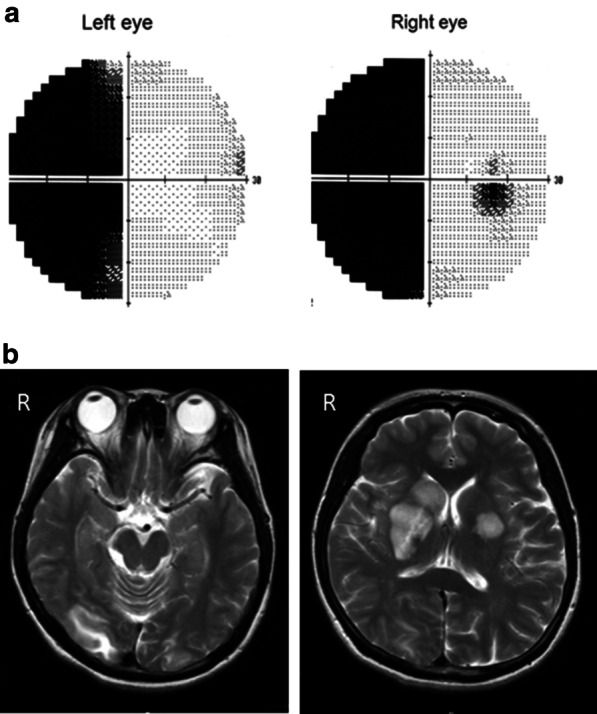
Visual field and magnetic resonance imaging before treatment. a Left homonymous hemianopsia was revealed. b MRI at 3 days after onset of the severe headache. T1-weighted-fluid-attenuated inversion recovery (T1-FLAIR) MRI revealed edema and infarction in the basal ganglions and right occipital lobe
